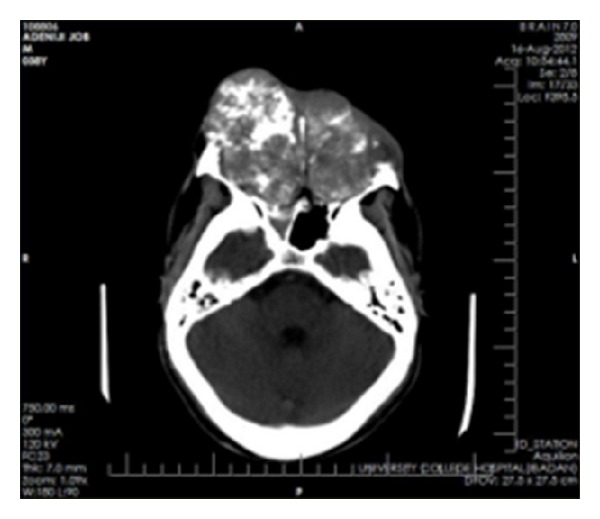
Axial CT showing the mass pushing out the orbital contents.
